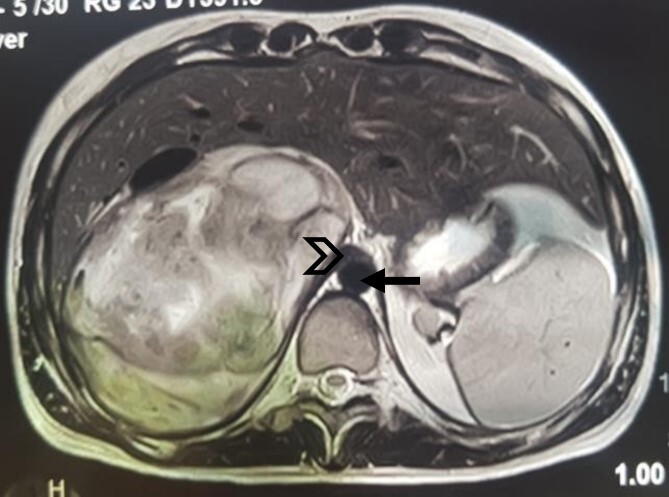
MRI, well-limited oval right adrenal mass, slightly heterogeneous mixed T2 signal (arrow: aorta; arrowhead: compressed IVC).
